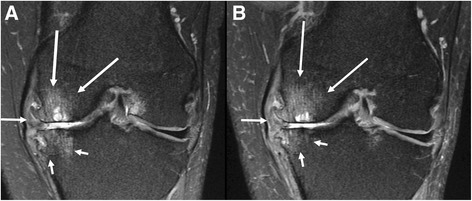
Coronal intermediate-weighted fat-suppressed MRI in treated patient at baseline (a) and 3 months (b) follow up show no improvement of cartilage damage in the medial tibiofemoral compartment (WORMS grade 6 at central medial femur; WORMS grade 5 at central medial tibia). There is a medial meniscal maceration/extrusion (arrow). There is a slight improvement of the subchondral bone marrow lesion/cysts at the central medial tibia (small arrows) and slight worsening of the subchondral bone marrow lesion/cysts at the central medial femur (long arrows). Note large medial osteophytes
